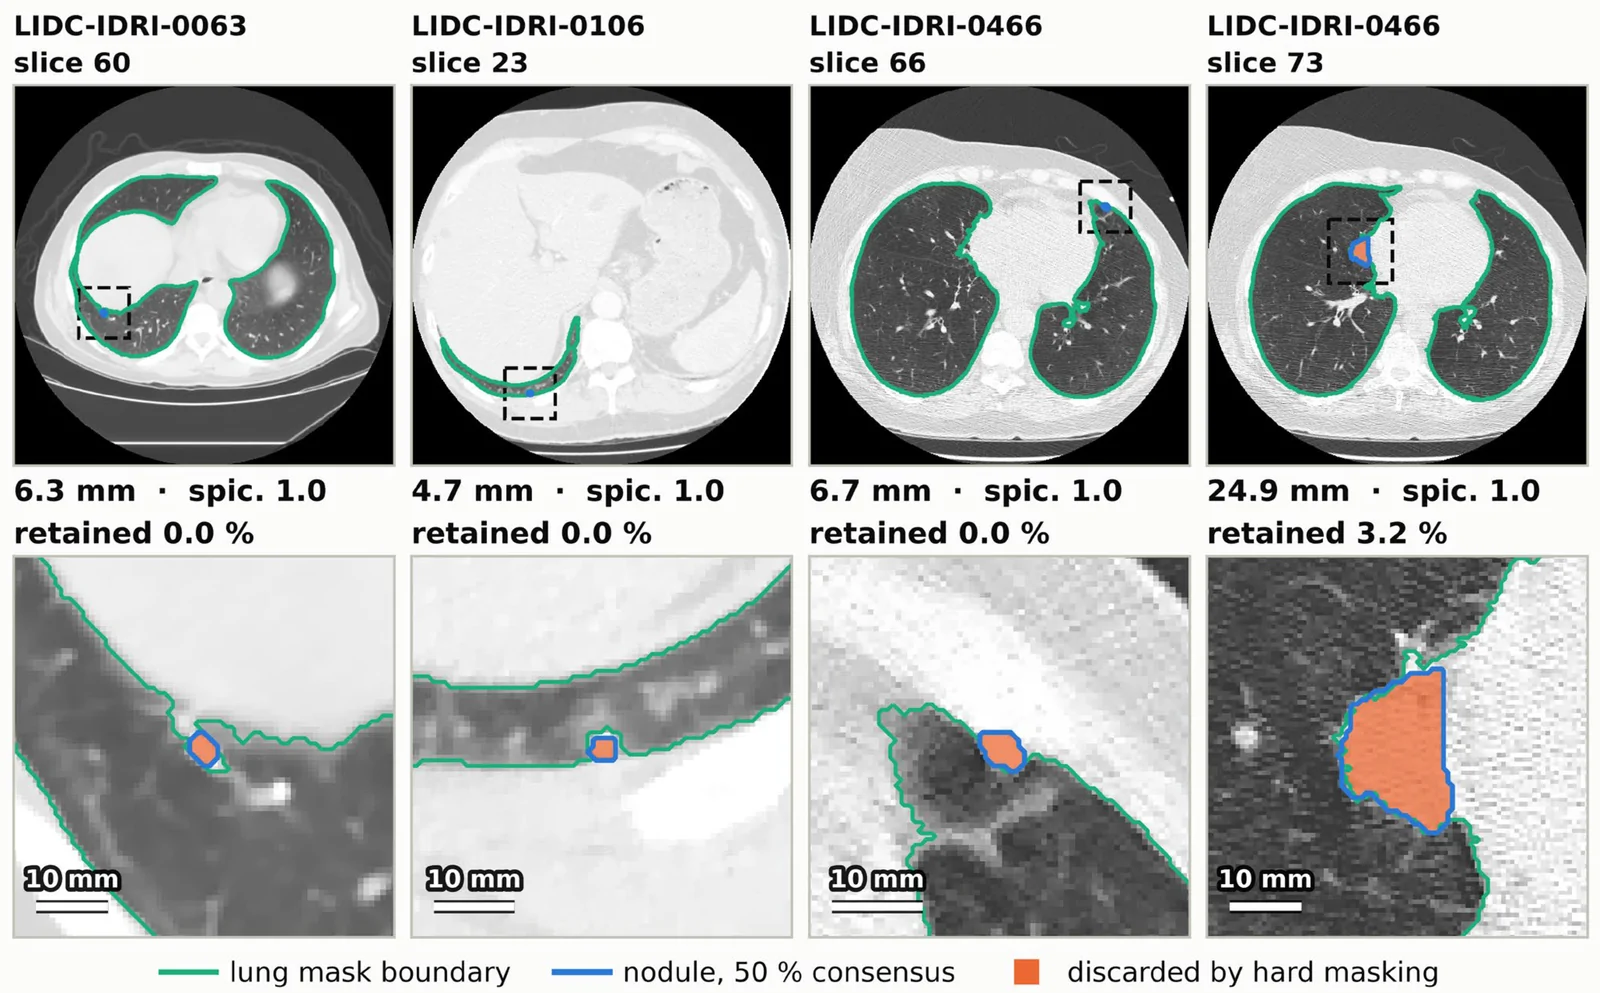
Using the lung mask to erase, rather than to locate, cuts into the lesion; the four worst hard-masking failures, on the CT. Top row, the full axial slice with the threshold lung mask boundary in green and the 50% consensus contour in blue, the dashed box marking the enlarged region; bottom row, that region enlarged, with orange marking the part of the lesion a hard mask discards. The window is identical in every panel (level −600 HU, width 1500 HU) and each scale bar is 10 mm. The two LIDC-IDRI-0466 panels are different nodules in the same series.
